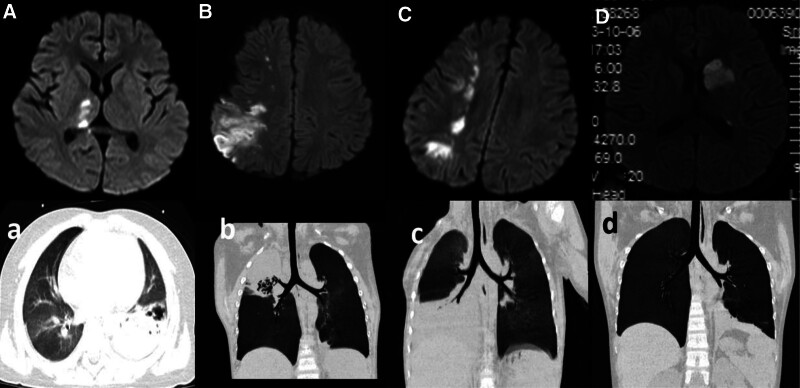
Brain MRI and chest CT findings of Cases. (A, B, C and D present images acquired during the DWI phase of patients with case 1, case 2, case 3, and case 4, respectively. a,b,c and d represent the chest CT of patients with case 1, case 2, case 3, and case 4, respectively). CT = computed tomography, DWI = diffusion weighted imaging, MRI = magnetic resonance imaging.
